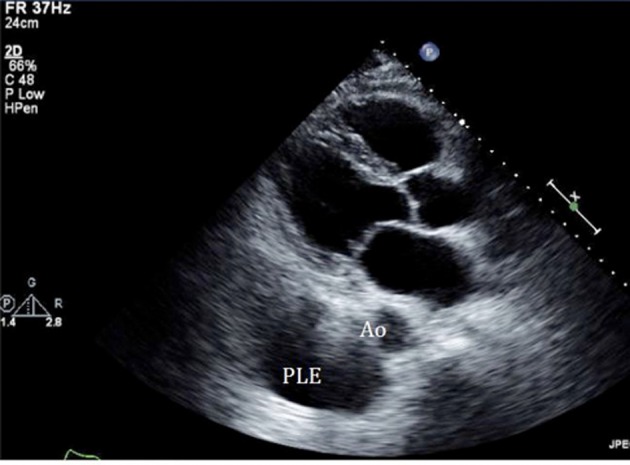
Echocardiogram performed after the pericardiocentesis showed resolution of the effusion, instead pleural effusion (PLE) becomes more evident as an echolucent space below the aorta (Ao).
